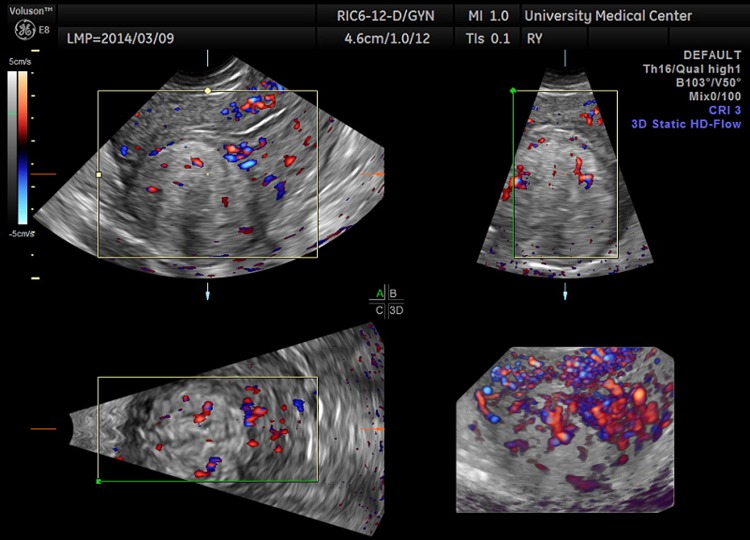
Orthogonal three-dimensional transvaginal ultrasound images of the uterus (with color Doppler overlay) showing vascularity both within and at the periphery of the mass.
